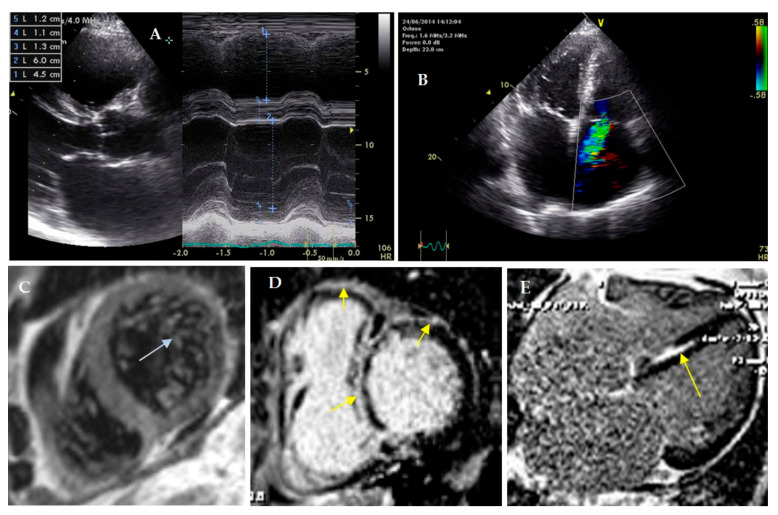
Cardiac visualization of proband. (A–C)—ultrasound cardiography. (C–E)—cardiac MRI with gadolinium. (A)—enlarged right ventricle and normal size of left ventricle; (B)—sever tricuspid regurgitation (Doppler). (C)—left ventricular noncompaction, ratio of noncompact and compact layers 3.5: 1, (D,E)—foci of late gadolinium enhancement in septum (subendocardial, intramyocardial), right ventricle and anterior wall of left ventricle (subepicardial).
